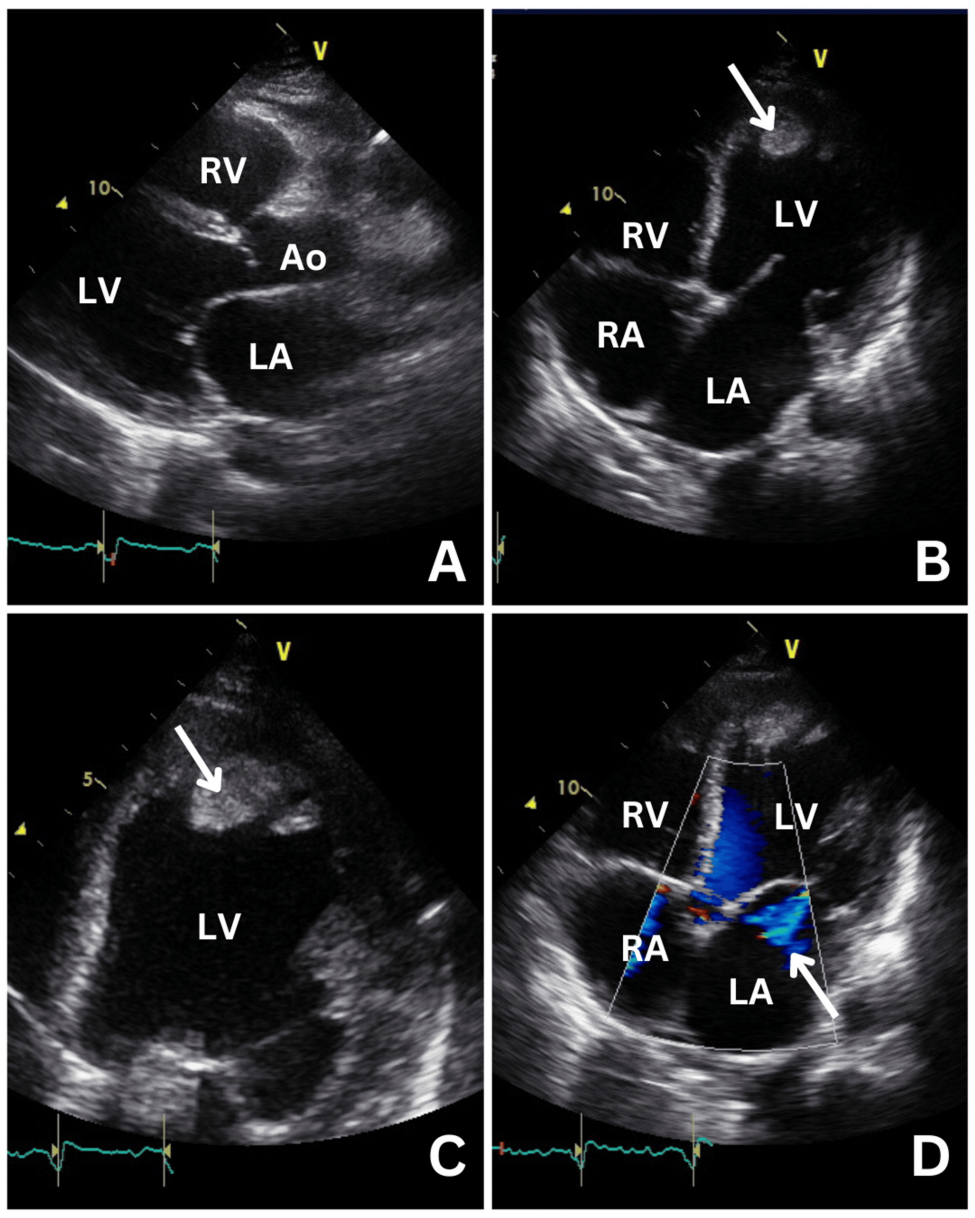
Transthoracic echo with labelled chambers. A. Parasternal long axis showing a dilated LV (left ventricle); B. apical four window showing LV apical thrombus (arrow) and dilated LV and LA (left atrium); C. focused view of LV apical thrombus (arrow); and D. functional mitral valve regurgitation colour Doppler eccentric jet (arrow) secondary to dilated LV. RV: right ventricle, RA: right atrium, Ao: aortic valve.
